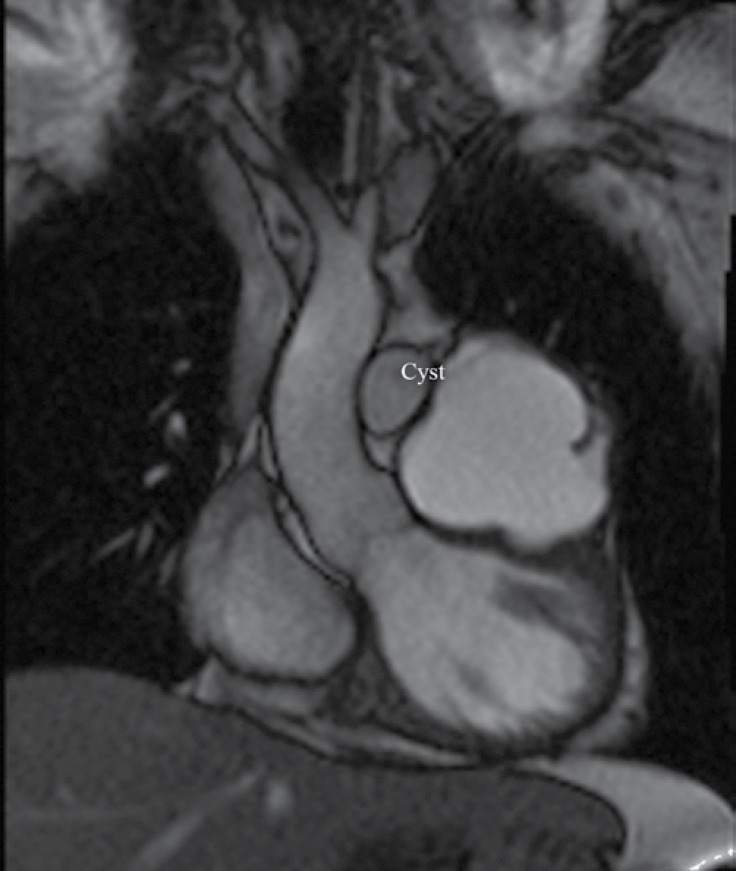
Cardiac magnetic resonance imaging reveals the close proximity of the cyst to vital the parts of the heart.
